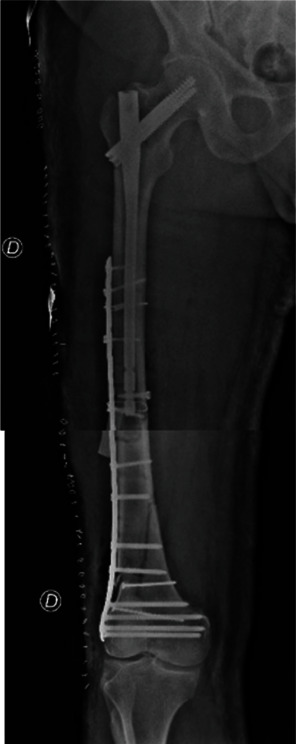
Post-surgical X-ray of the right femur after osteosynthesis with proximal femoral nail and distal femur anatomical plate.
